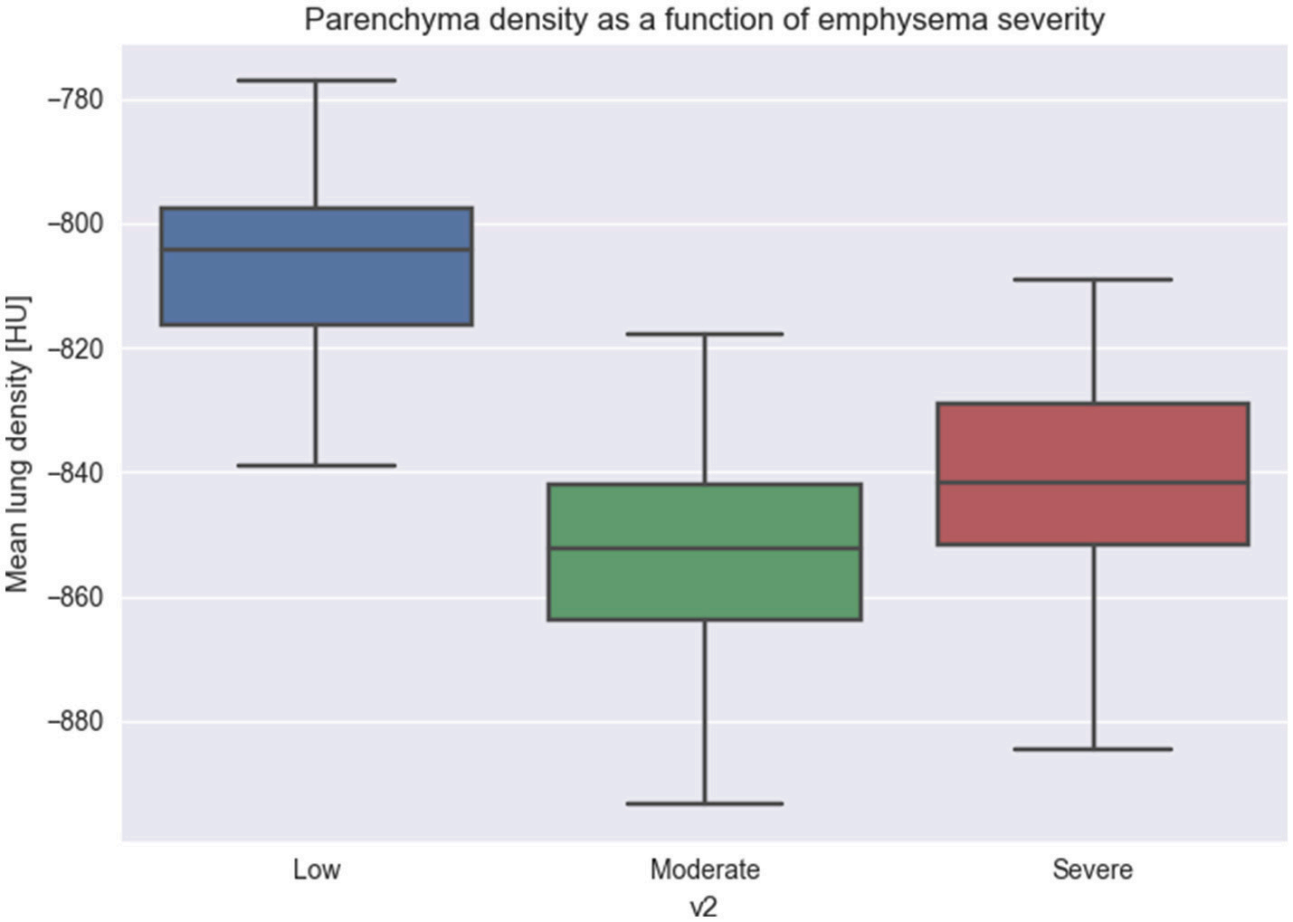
Relation between Mean Lung Density and emphysema progression. We used Mean Lung Density (MLD) to quantify patches belonging to the three emphysema classification levels. The figure shows that emphysema progression is associated with a mean lowering of the MLD values, due to the destruction of parenchyma and the diminishing of the CT attenuation value.
